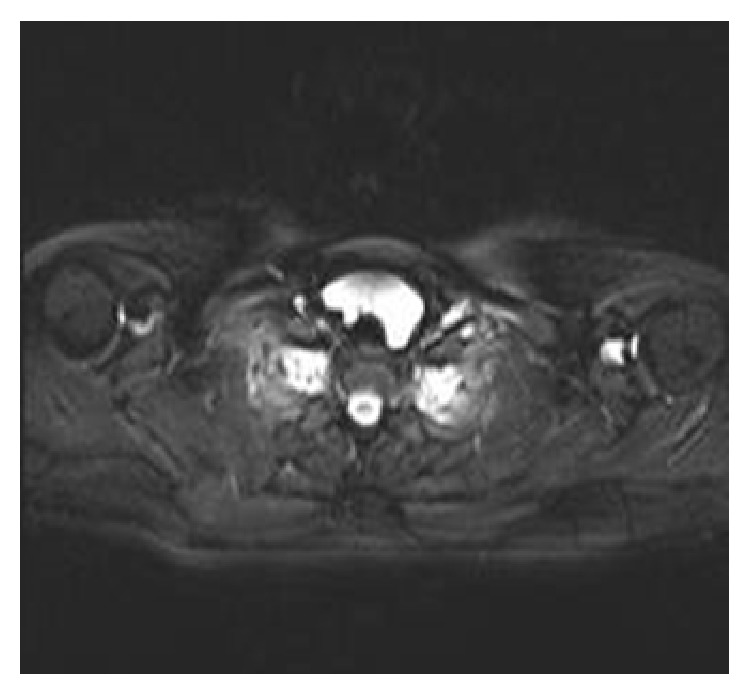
T2-weighted axial MRI showing the lesion's pretracheal position.
